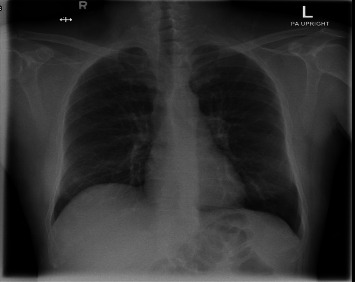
Chest X-ray: showing unremarkable chest X-ray on admission.
